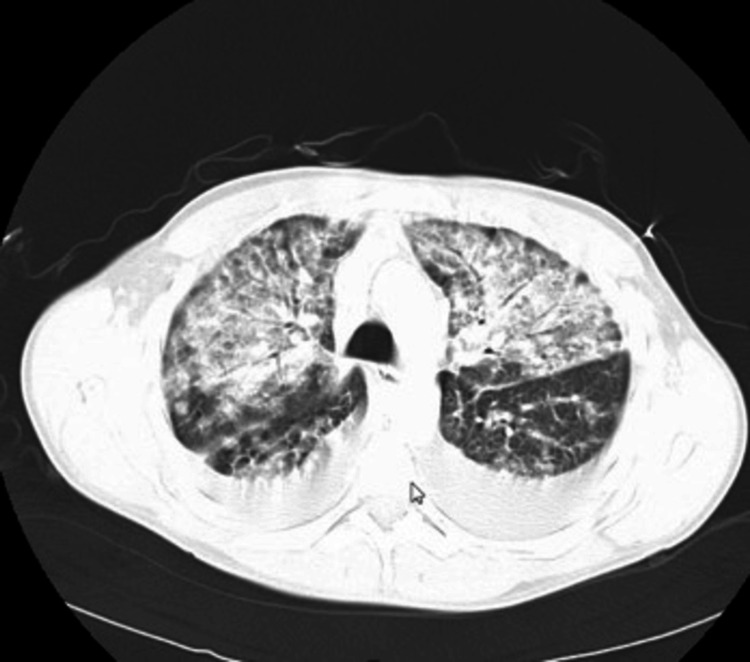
CT of the chest with IV contrast showing progression of the bilateral diffuse peribronchovascular and subpleural ground-glass opacities, with progression of consolidation. Bilateral small to moderate pleural effusions were noted, with unchanged mediastinal or hilar lymphadenopathy. No central airway obstruction was found.
